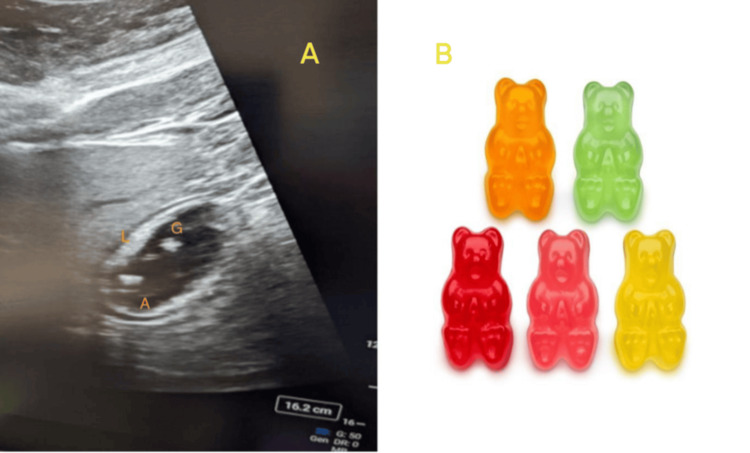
(A) Gastric ultrasound demonstrating the assessment of gastric contents. The sonographic image shows echogenic solid structures consistent with gummy bears within the stomach, along with the gastric antrum and left lobe of the liver. (B) Gummy bears L: left lobe of the liver; G: gummy bears; A: gastric antrum
